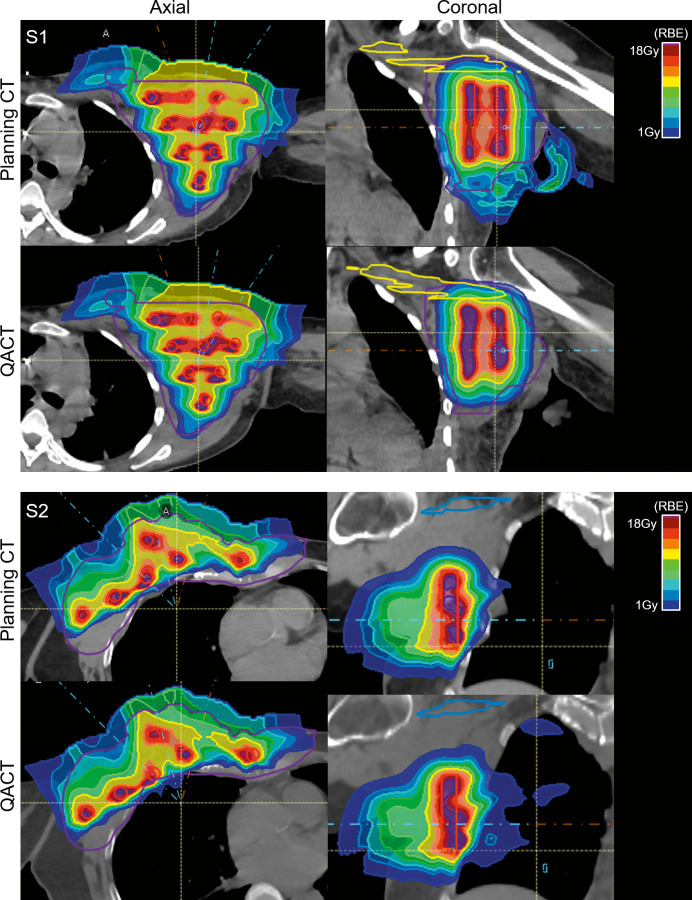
PBS-GRID plans for 2 patients (S1 and S2) enrolled onto a prospective phase 1 clinical trial evaluating the feasibility of proton GRID for the treatment of bulky tumors. PBS GRID with 3-cm center-to-center spacing was used. Plan computed on the planning CT (top) and on the QACT 1 day prior to treatment (bottom) shown in the axial and coronal planes. S1: purple contour indicates GTV, yellow contour indicates brachial plexus (the dose limiting organ), and red indicates the GRID targets. S2: purple contour indicates the GTV, blue contour indicates the brachial plexus, and red contours indicate the GRID targets. Abbreviations: CT, computed tomography; GRID, spatially fractionated radiation therapy; GTV, gross tumor volume; PBS, pencil-beam scanning; QACT, quality assurance CT.
